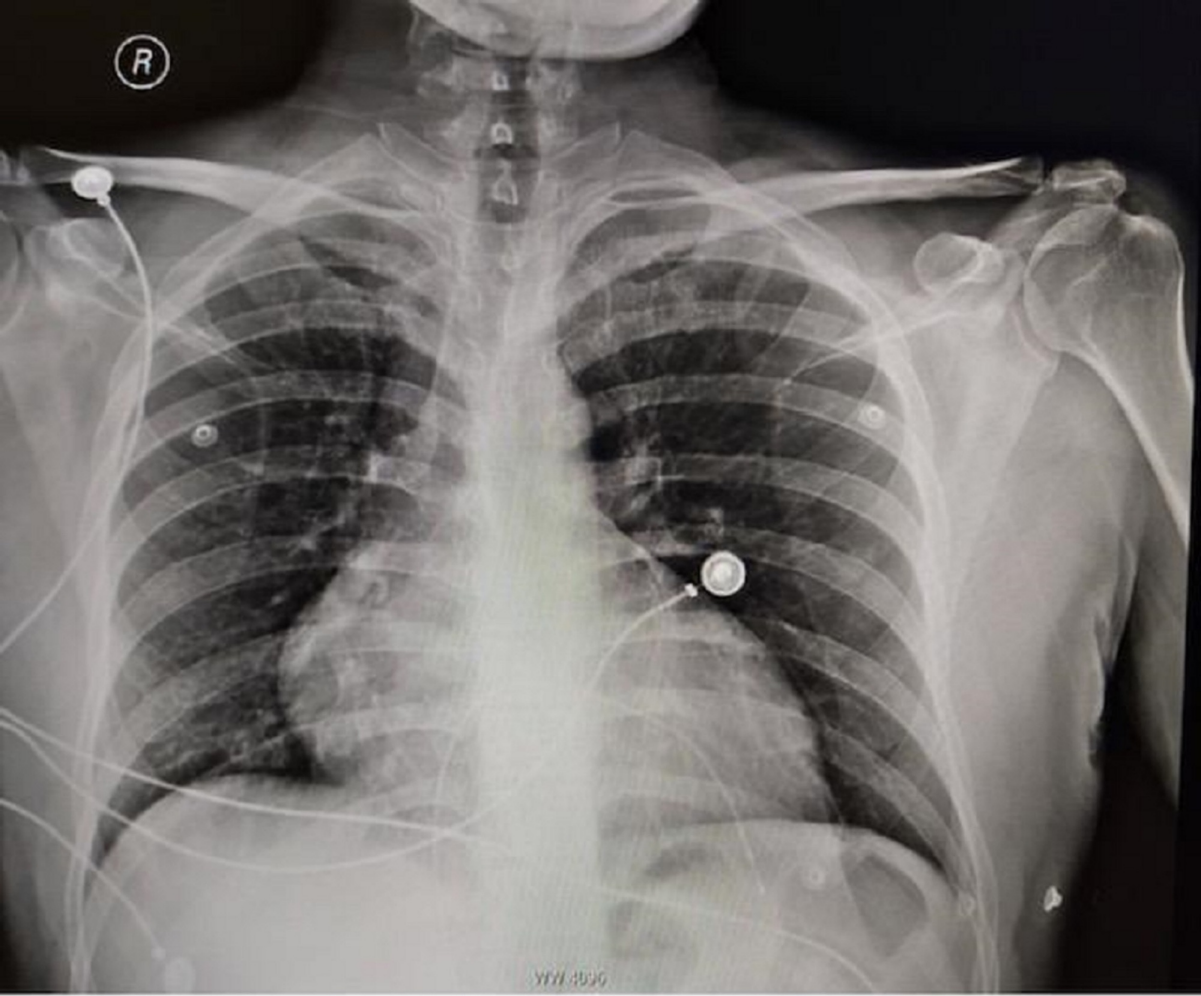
A chest x-ray revealed normal findings with normal cardiac shadow and lung fields.
